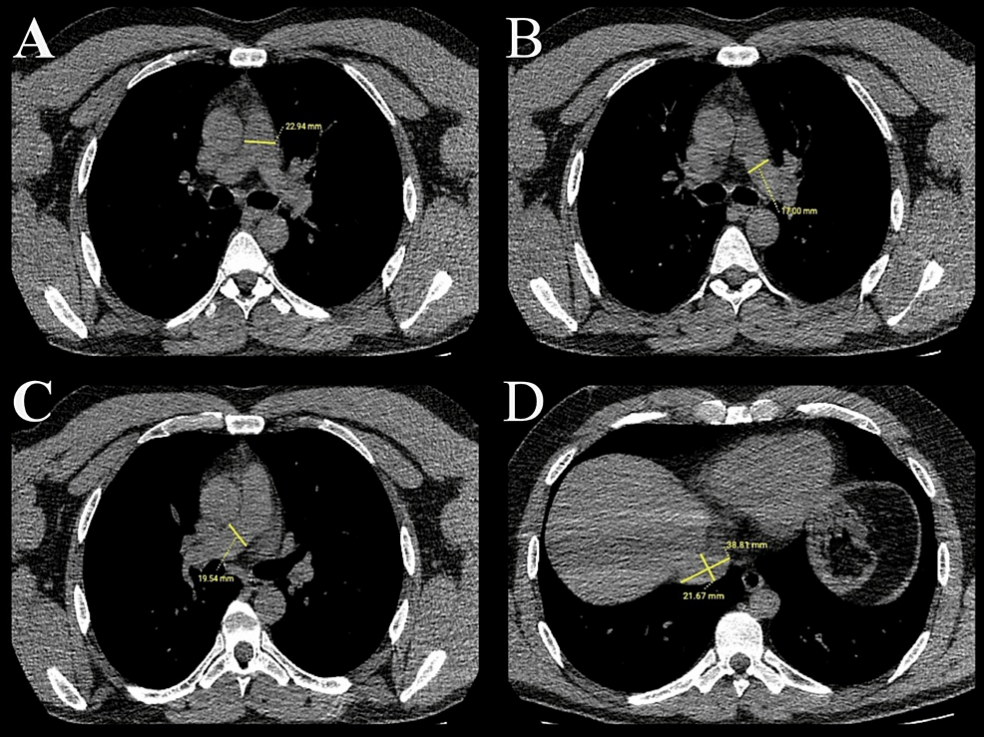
Measuring the diameters of vascular structures. In axial non-contrast CT scans, A shows the measurement of the main pulmonary artery diameter. B shows the measurement of the left pulmonary artery diameter. C shows the measurement of the right pulmonary artery diameter. D shows the measurement of the transverse and anteroposterior inferior vena cava diameter.
